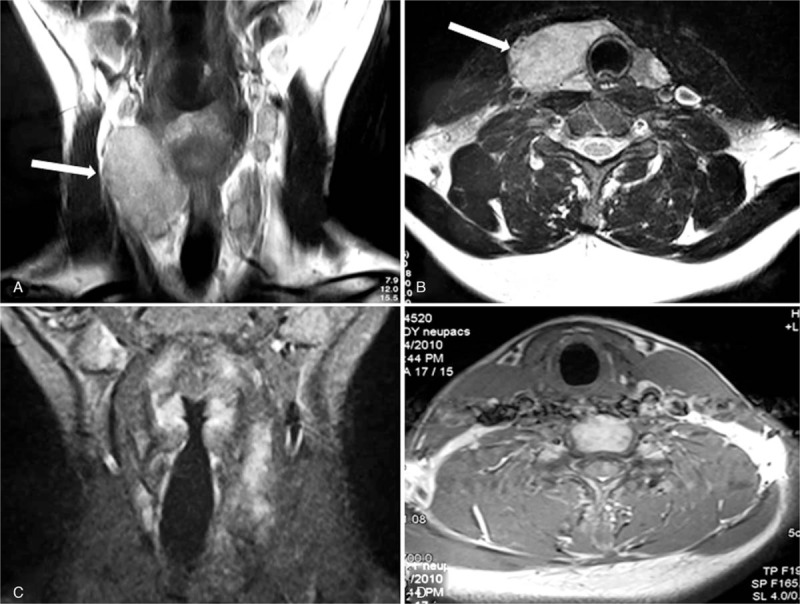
Magnetic resonance image of thyroid showed the obvious enlargement on the right lobe of thyroid in the coronal plane (A) and cross section (B) before surgery; It showed the right lobe of thyroid has no lesions four years (C and D) after surgery.
